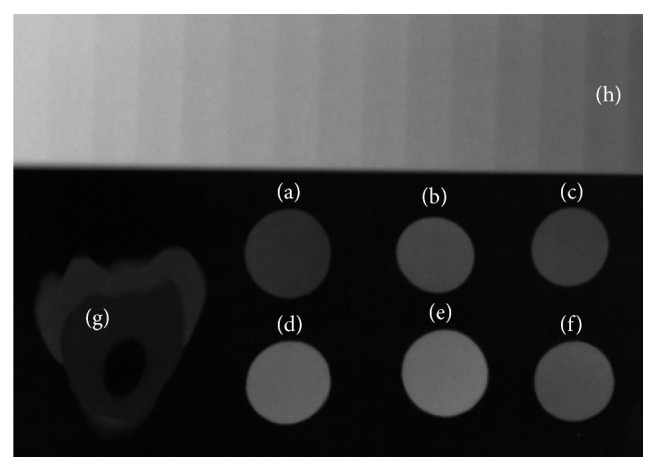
Radiographic image of resin samples ((a) P90, (b) Z350, (c) Z350 flow, (d) Tetric Ceram, (e) TPH, (f) SDR), slice of human tooth (g), and aluminum step-wedge (h).
